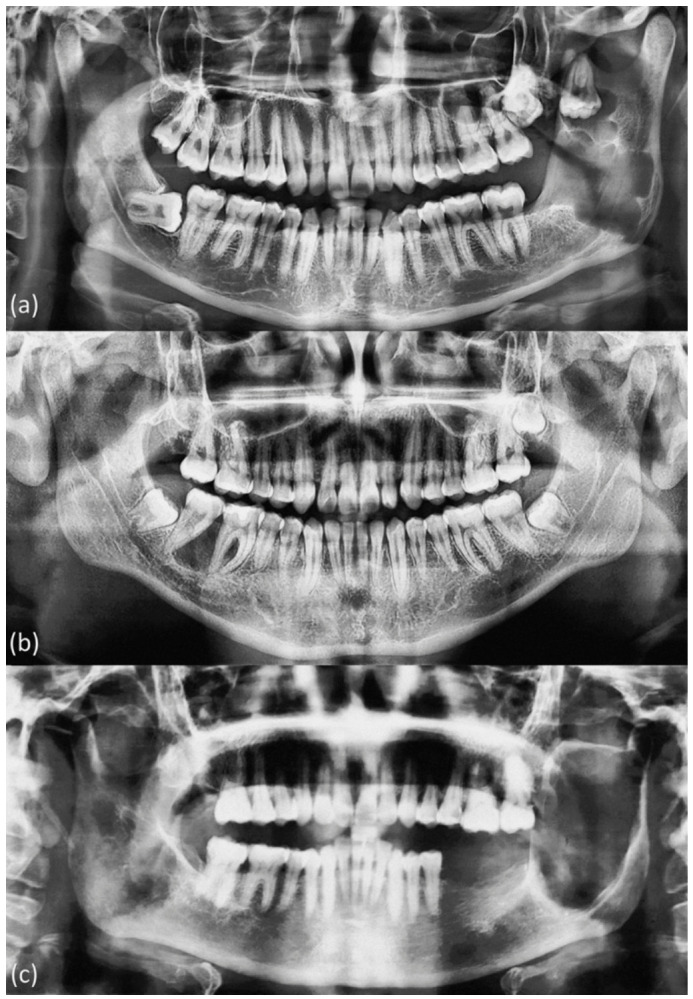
Radiographic images of the odontogenic keratocyst. (a) Panoramic radiograph showing a multilocular radiolucent lesion associated with an impacted third molar displaced to the level of the coronoid process, at the angle and ascending ramus of the left mandible. (b) Panoramic radiograph showing a unilocular radiolucent lesion at the interradicular and apical level of the right mandibular first and second molars. (c) Panoramic radiograph showing a unilocular lesion that was radiographically diagnosed as a residual cyst, extending throughout the entire left ascending mandibular ramus.
